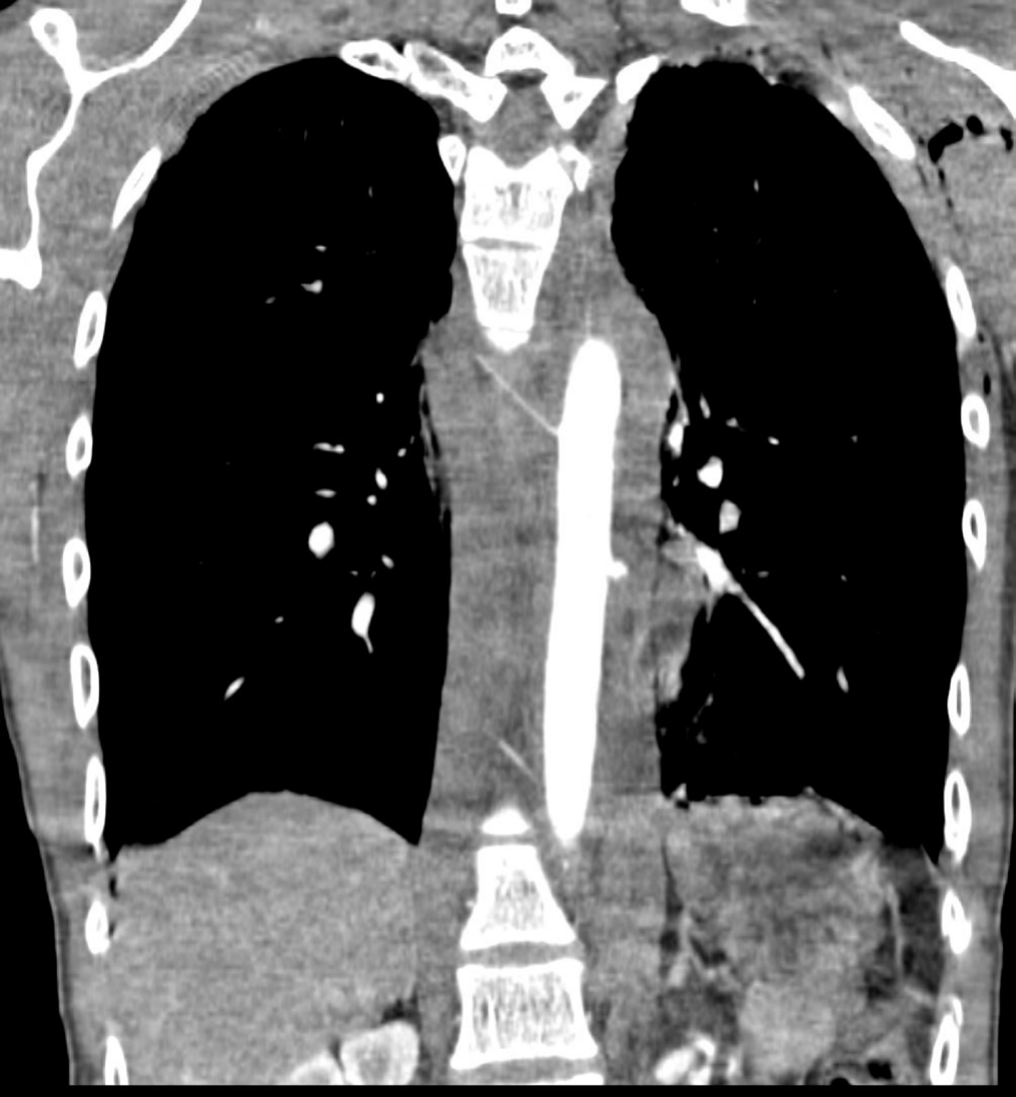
Coronal angio-CT image showing contrast leakage from the thoracic aorta.
